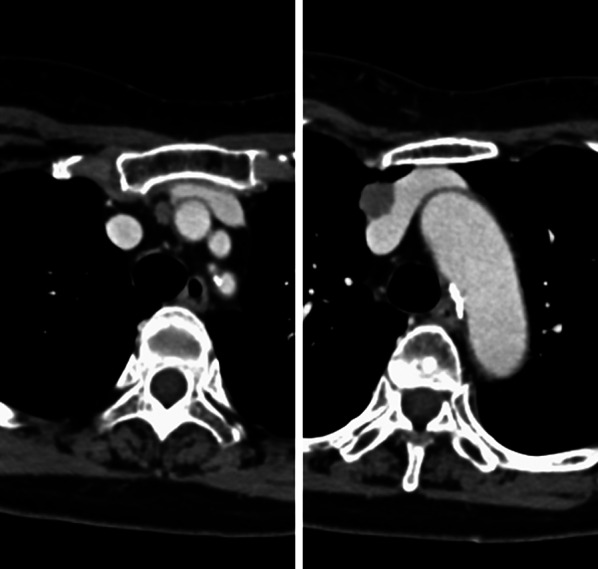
Chest computed tomography (CT) revealed a recurrent lesion in the anterior mediastinum
